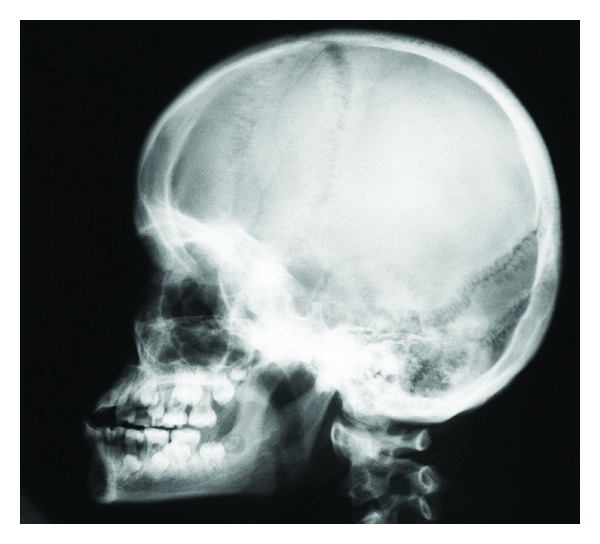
Profile radiograph of a girl with Down syndrome, aged 8 years 6 months. Note the enlarged thickness of the skull, absence of the nasal bone, deviations in the upper contour of the anterior wall of the sella turcica, malformations of the cervical spine, and maxillary retrognathia. Deviations of the skeleton occur in several craniofacial fields.
